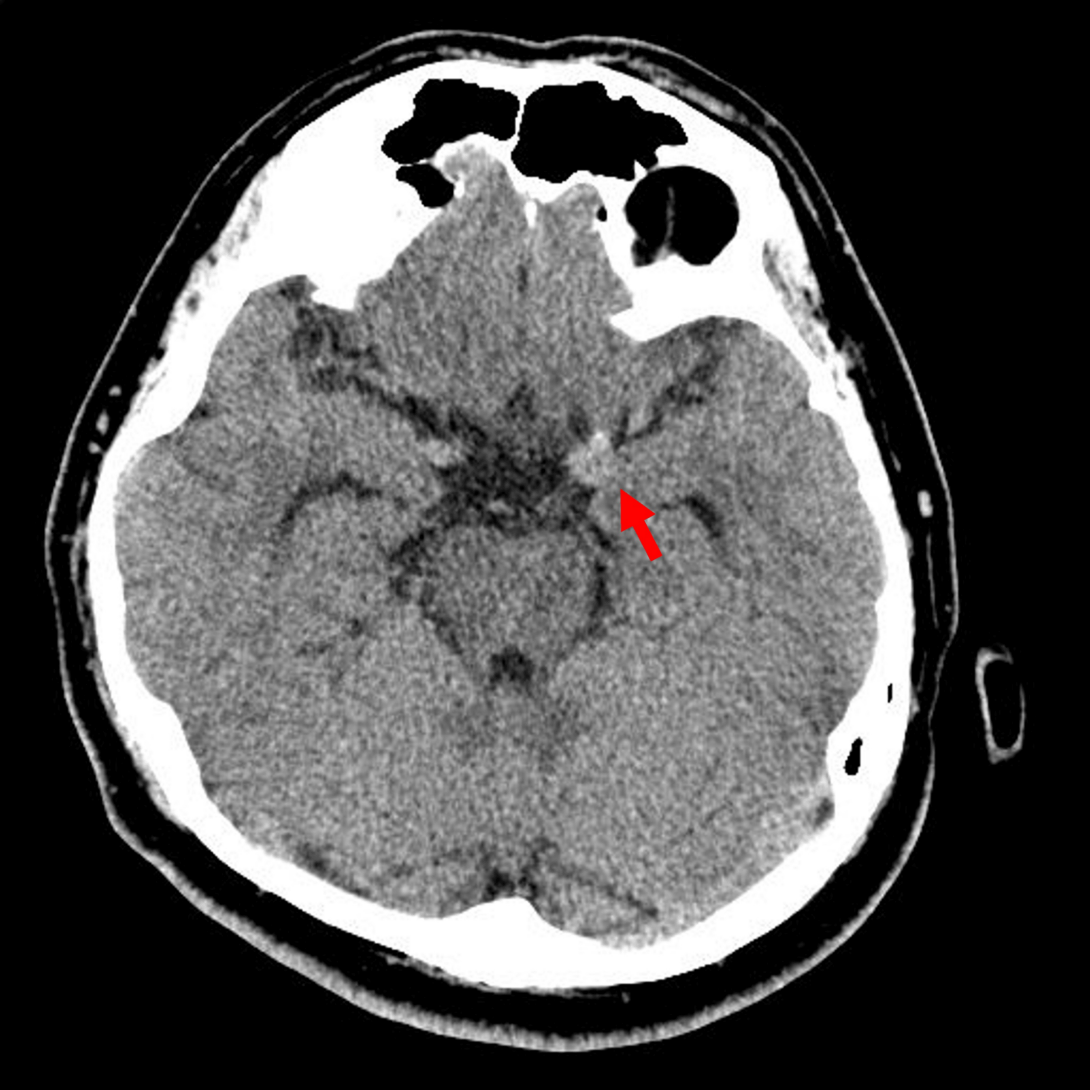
Initial non-contrast head CT findings Initial non-contrast head computed tomography (CT) scan demonstrating a hyperdense lesion (arrow) suspicious for a distal left internal carotid artery (ICA) aneurysm. No evidence of subarachnoid hemorrhage (SAH) is seen.
